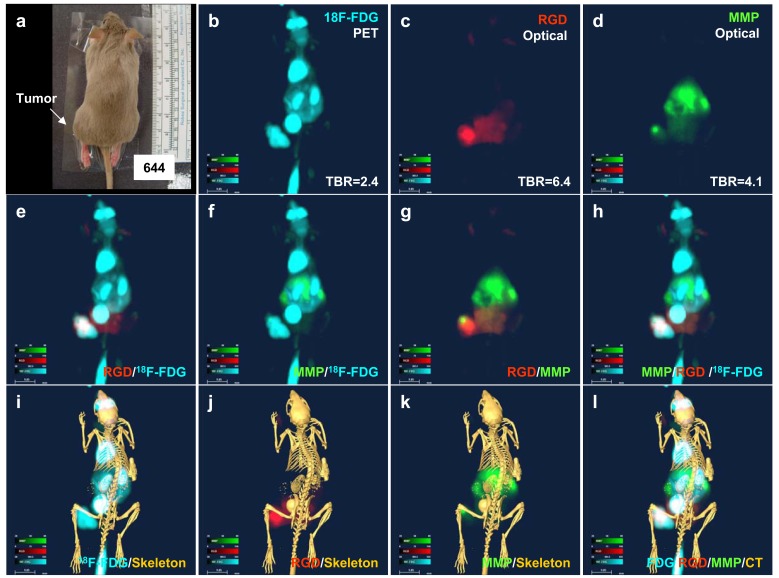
In vivo* imaging of a liver cancer xenograft. (a) The color image shows the tumor location. (b) ^18^F-FDG PET imaging
